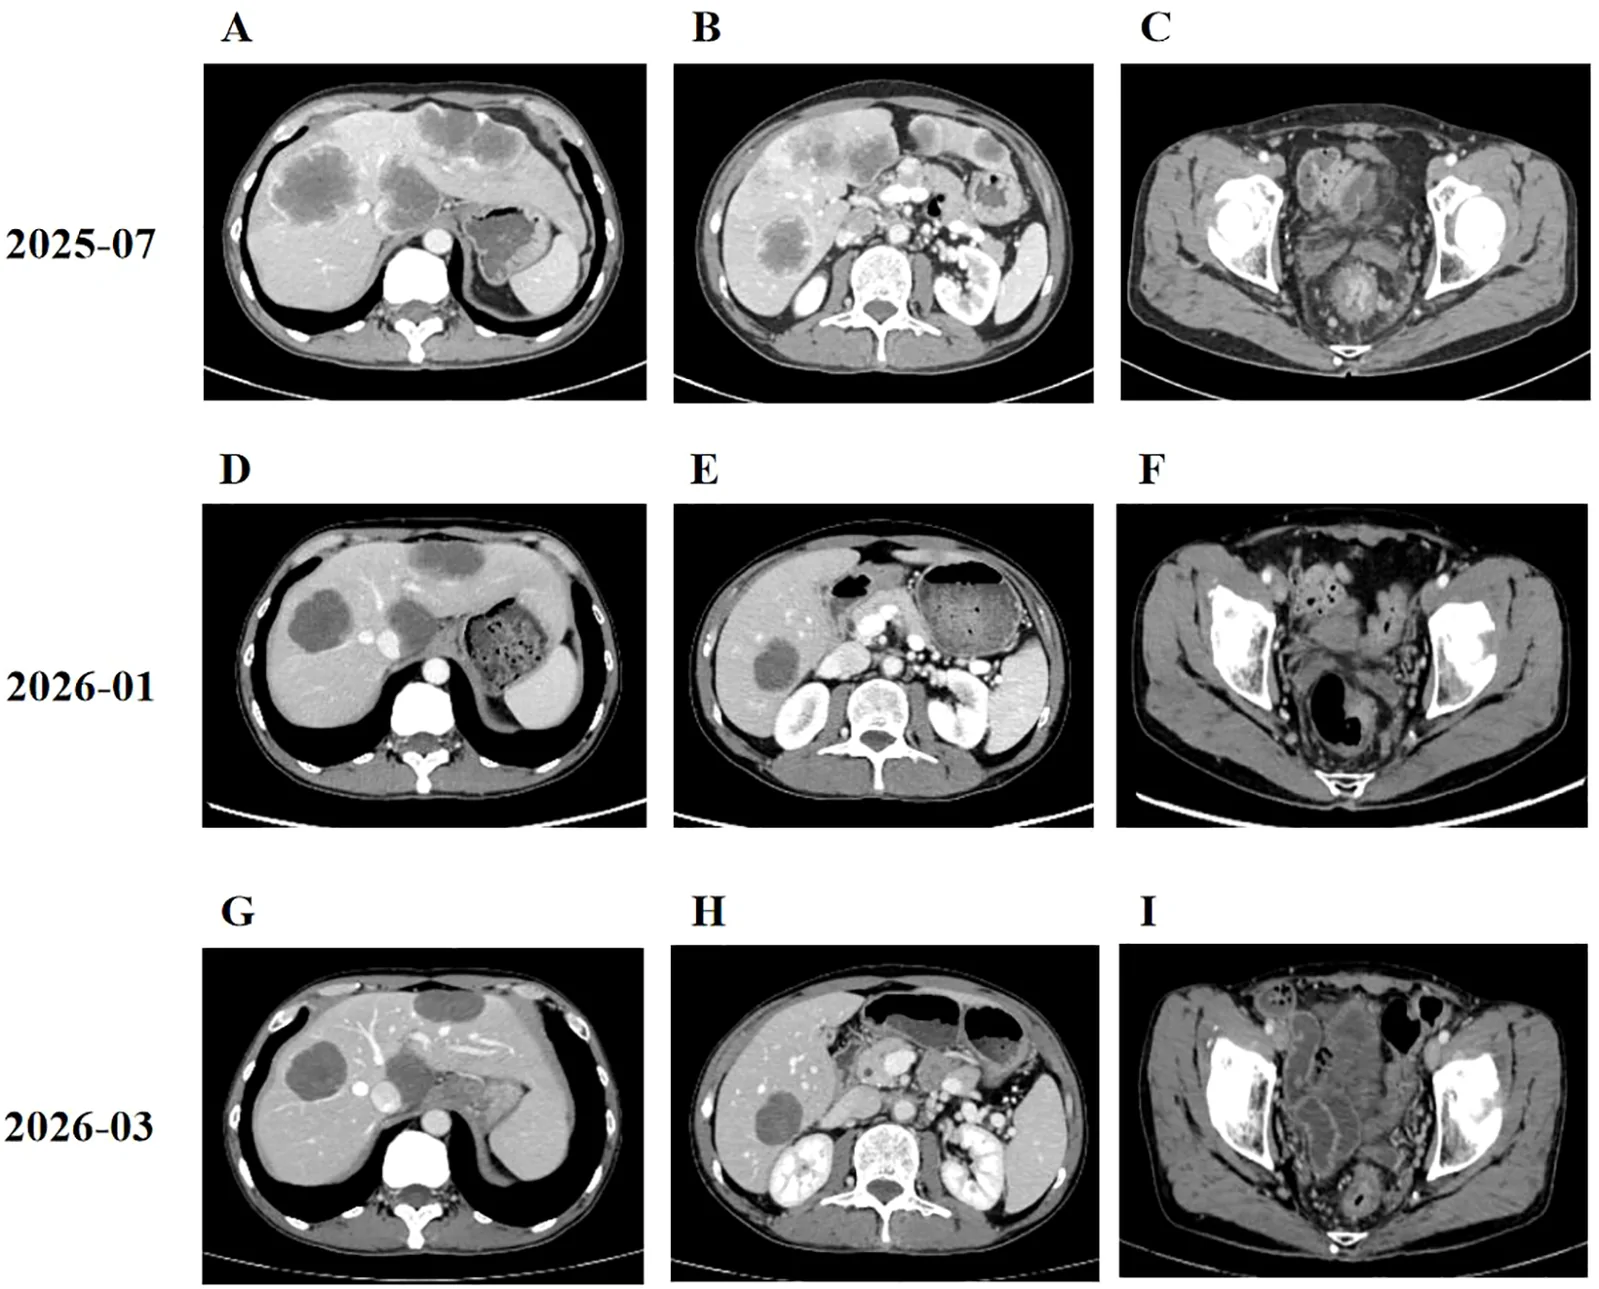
Imaging examination of liver mass and primary lesion. Abdominal CT showing the radiological response to therapy. (A–C) advanced rectal cancer with liver and lymph nodes metastases at the initial diagnosis. (D–F) partial response was achieved after six cycles of CAPOX+Bevacizumab treatment. (G–I) continued partial response after four cycles of FOLFOX+Bevacizumab+Zanidatamab treatment.
